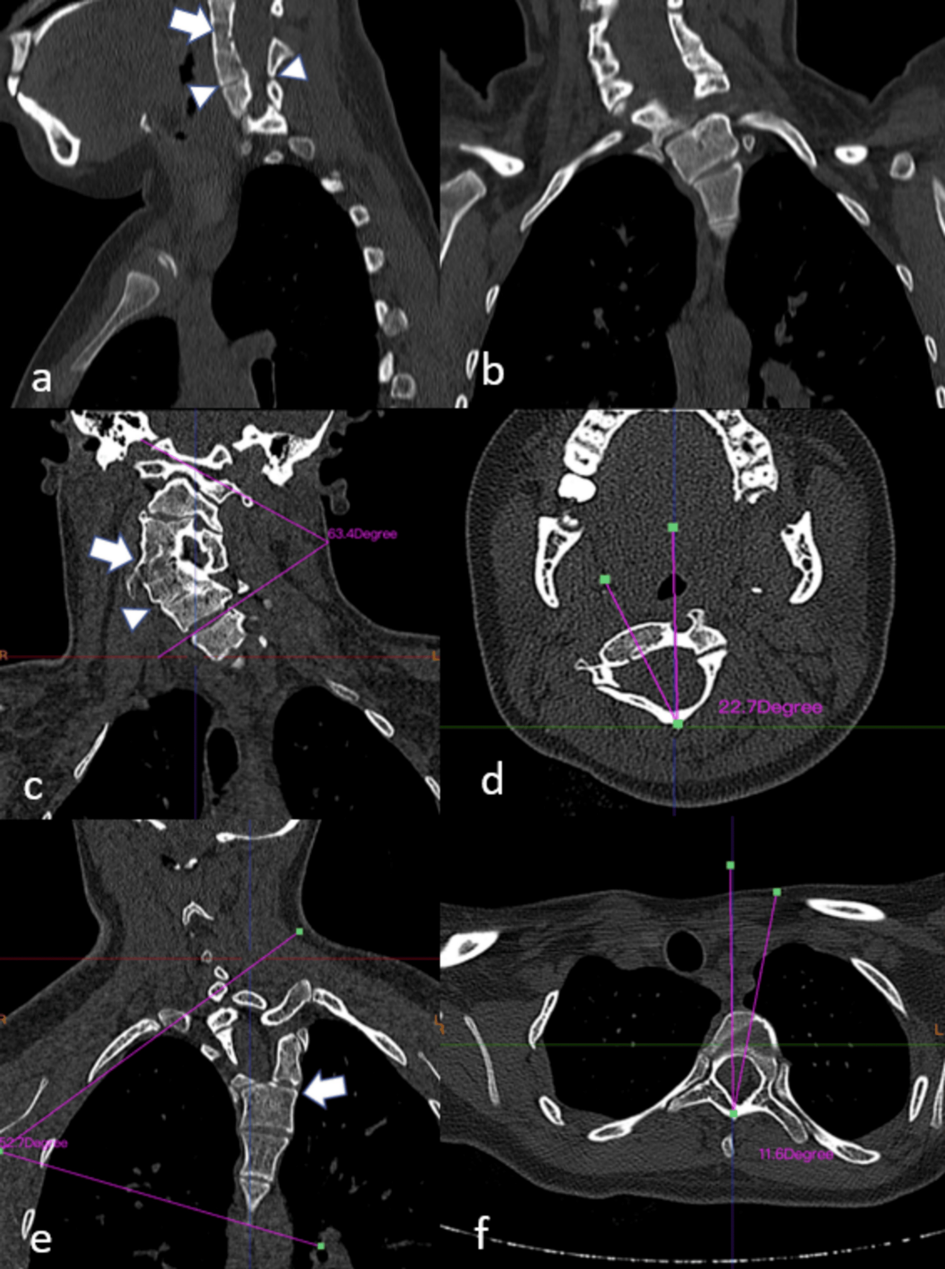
Cervical computed tomography (CT) scan showing total fusion of the C3-C4-C5 vertebral bodies with partial posterior fusion of the C5-C6 vertebral bodies. Sagittal view (a), coronal view (b), cervical scoliosis (arrow) with a Cobb angle of 63.4°, partial posterior fusion of the C5-C6 vertebral bodies (arrowhead) (c), apical cervical vertebra rotation in the axial plane of 22.7° (d), thoracic scoliosis (arrow) with a Cobb angle of 52.7° (e), and apical dorsal vertebra rotation in the axial plane of 11.6°(f).
